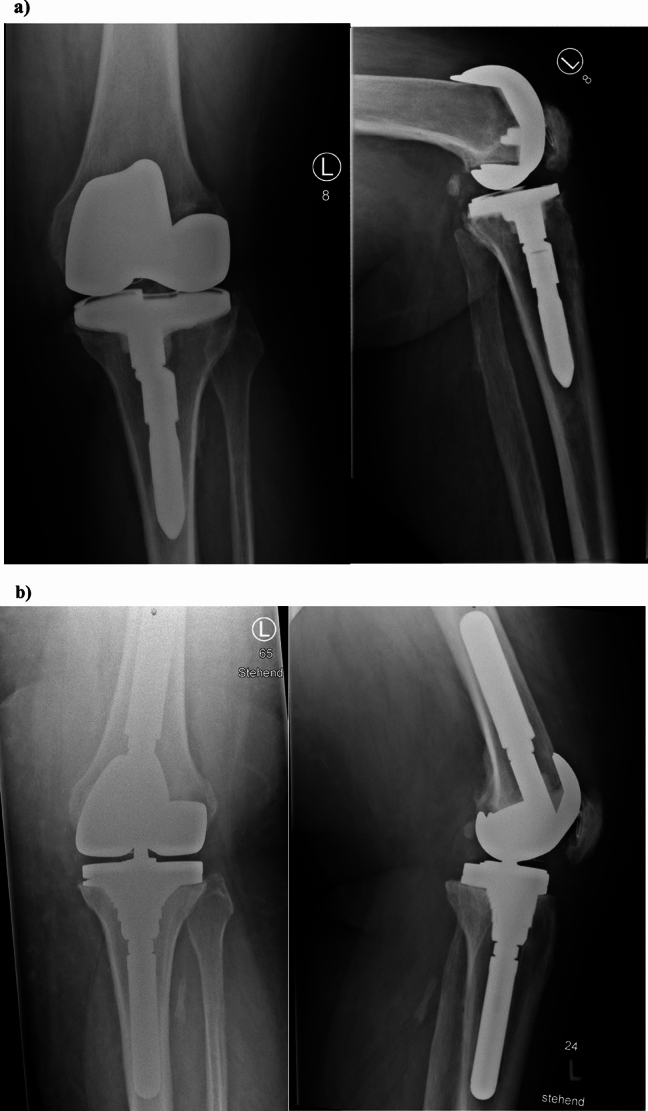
Case 2 shows a morbidly obese, 52 year-old male patient with a multidirectional instability KDC B and aseptic loosening of the femoral and tibial component. a Properative x-rays anterior–posterior view on the left, lateral view on the right. Unconstrained, cruciate-retaining prosthesis with a tibial modular un-cemented stem. Radiolucent lines can be observed on the femur and around the tibial stem. b Postoperative x-rays anterior–posterior view on the left and lateral view on the right. After removal of the implant in this single-stage exchange the femur displayed a KDC 1 defect. The small epiphyseal defects could be augmented with PMMA. The removal of the tibial component resulted in a KDC 2 defect which was treated with a metaphyseal sleeve and an un-cemented modular stem. Due to the persistent instability after anatomic reconstruction of the joint line (KDC B) a rotating-hinge prosthesis was chosen
